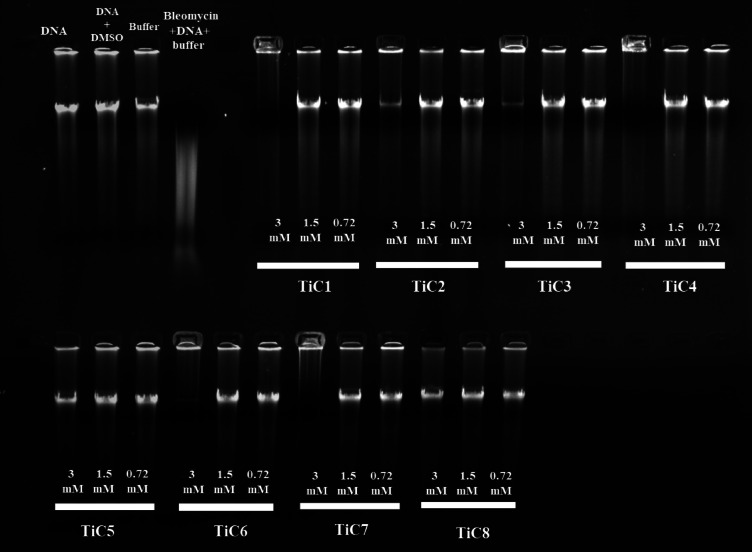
Agarose gel electrophoresis of CT-DNA with TiC1-TiC8 (1) CT-DNA in PBS buffer; (2) CT-DNA in PBS buffer + DMSO; (3) PBS; (4) CT-DNA in PBS buffer + bleomycin; (5) Lanes 5–28 CT-DNA+ (3, 1.5 and 0.75 mM) TiC1-TiC8.
